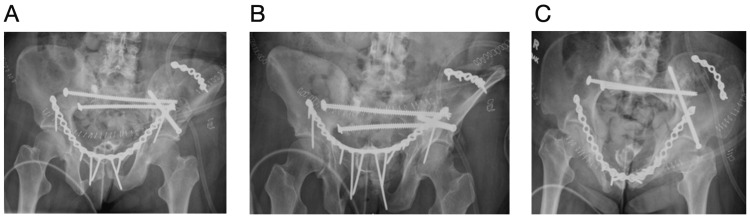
Post op radiographs Images A (AP pelvis) , B (pelvic outlet), and C (pelvic inlet) demonstrate the status post fixation for multiple pathological fragility fractures. The iliac crest fracture was stabilized with a 6-hole plate. A 20-hole plate was used to stabilize the anterior pelvis. There are three cannulated screws, one through the LC-2 corridor, one in the S1 corridor, and one in the S2 corridor.
